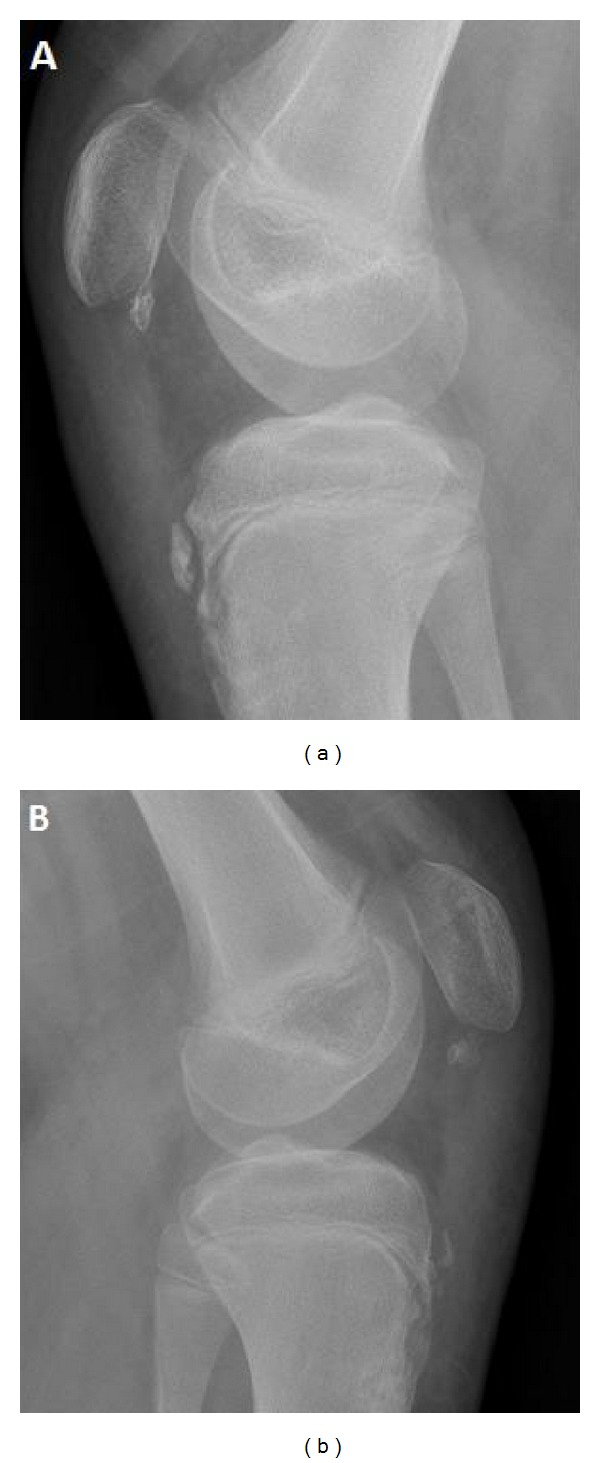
Postoperative radiographs of (a) right and (b) left knees show a decrease in patellar height.
